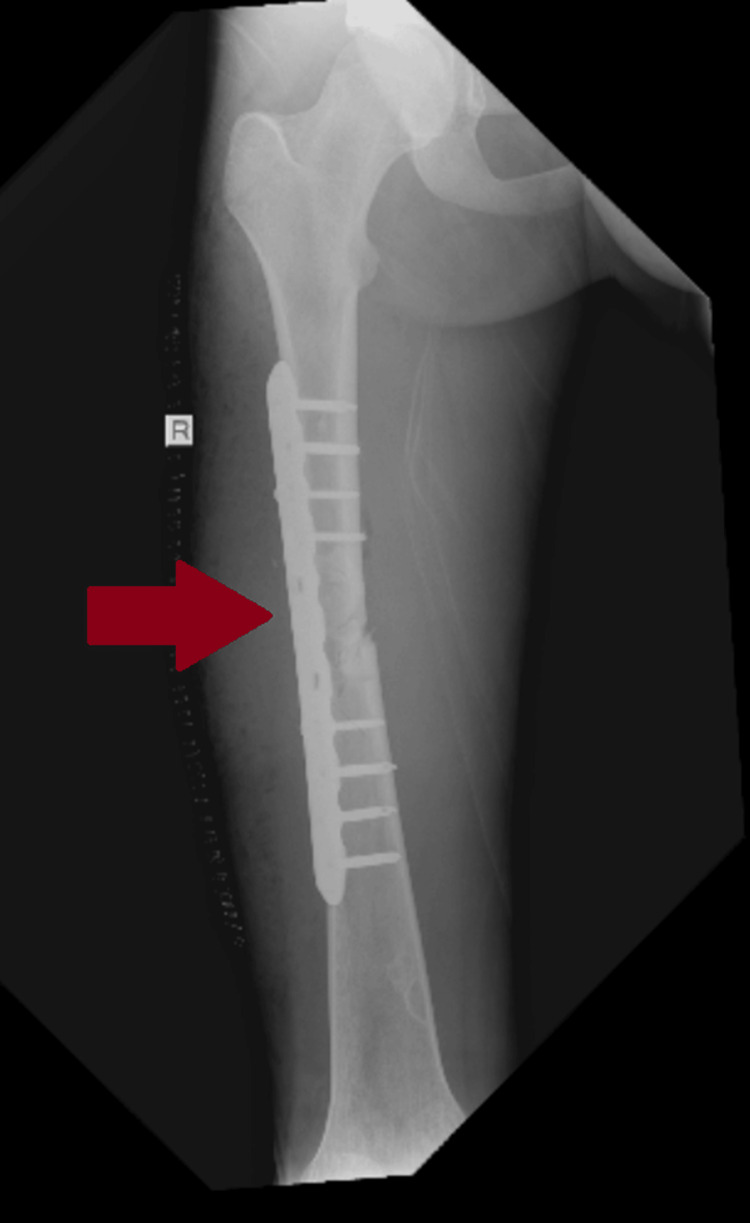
Immediate anteroposterior postoperative X-ray after fixation of the diaphyseal femur fracture with a plate
